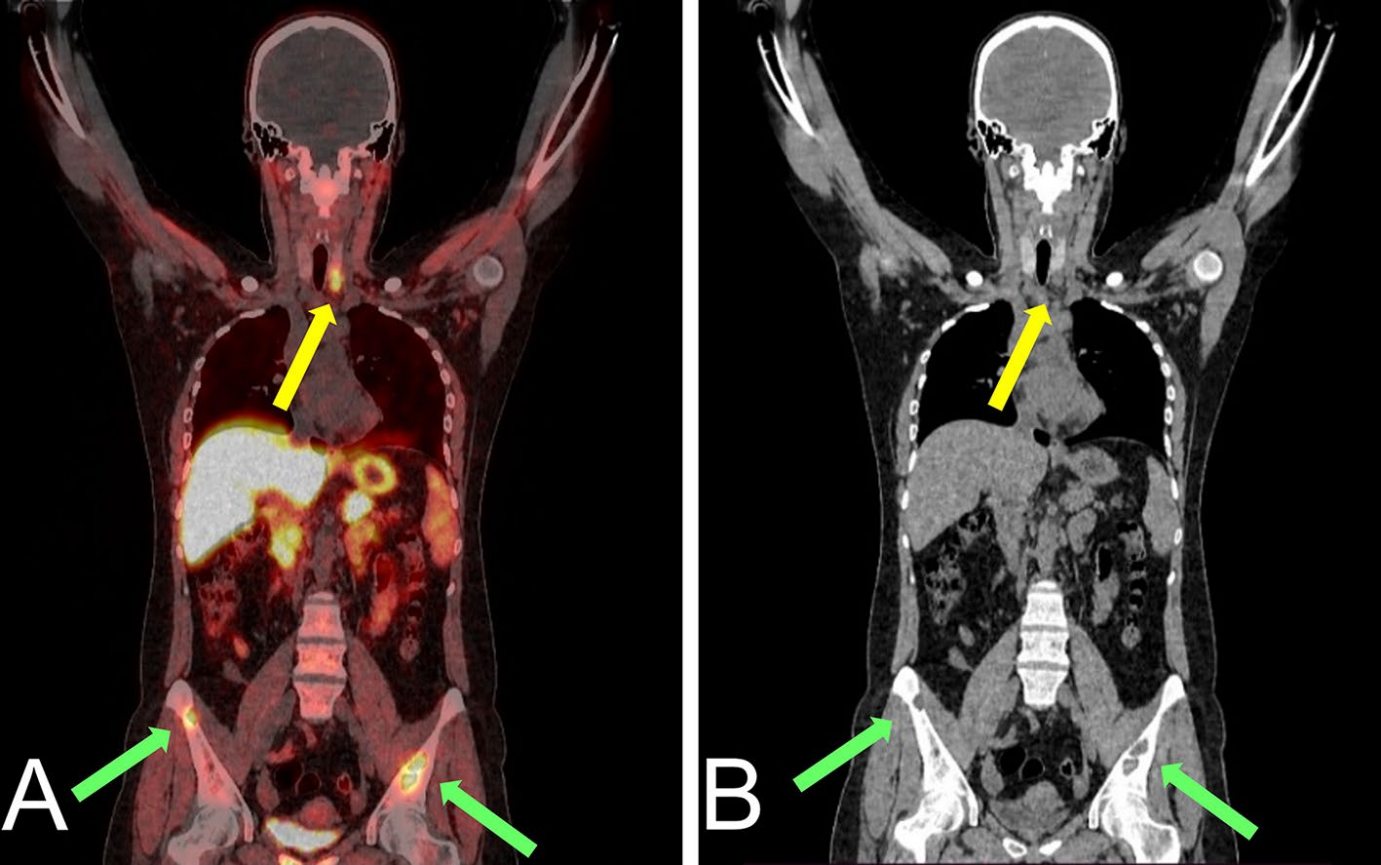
Coronal [^18^F]fluorocholine PET/CT images of hyperfunctioning parathyroid tissue (yellow arrows) on lower left parathyroid lodge and avid lesions involving iliac bone bilaterally (green arrows) (SUVmax 4.5 on the left and 7.9 on the right) (A) and CT coregistration (B)
